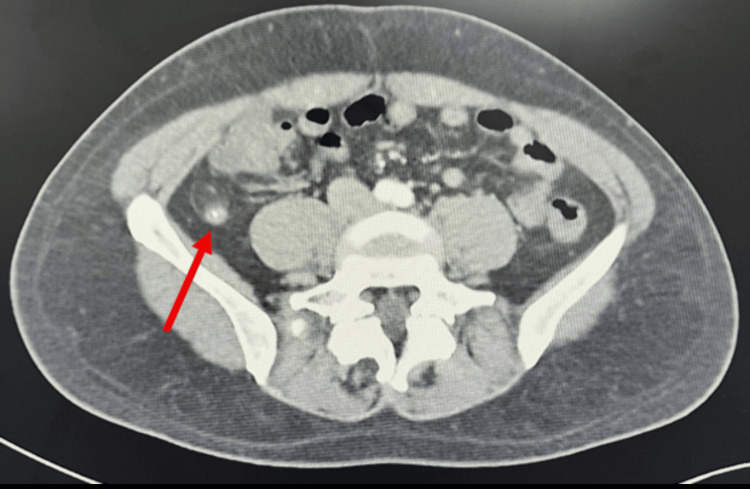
CT showing an appendicolith at the distal appendiceal tip. Axial contrast-enhanced CT image of the abdomen demonstrates a hyperdense appendicolith located at the distal tip of the appendix (arrow), with associated inflammatory changes consistent with acute appendicitis.
